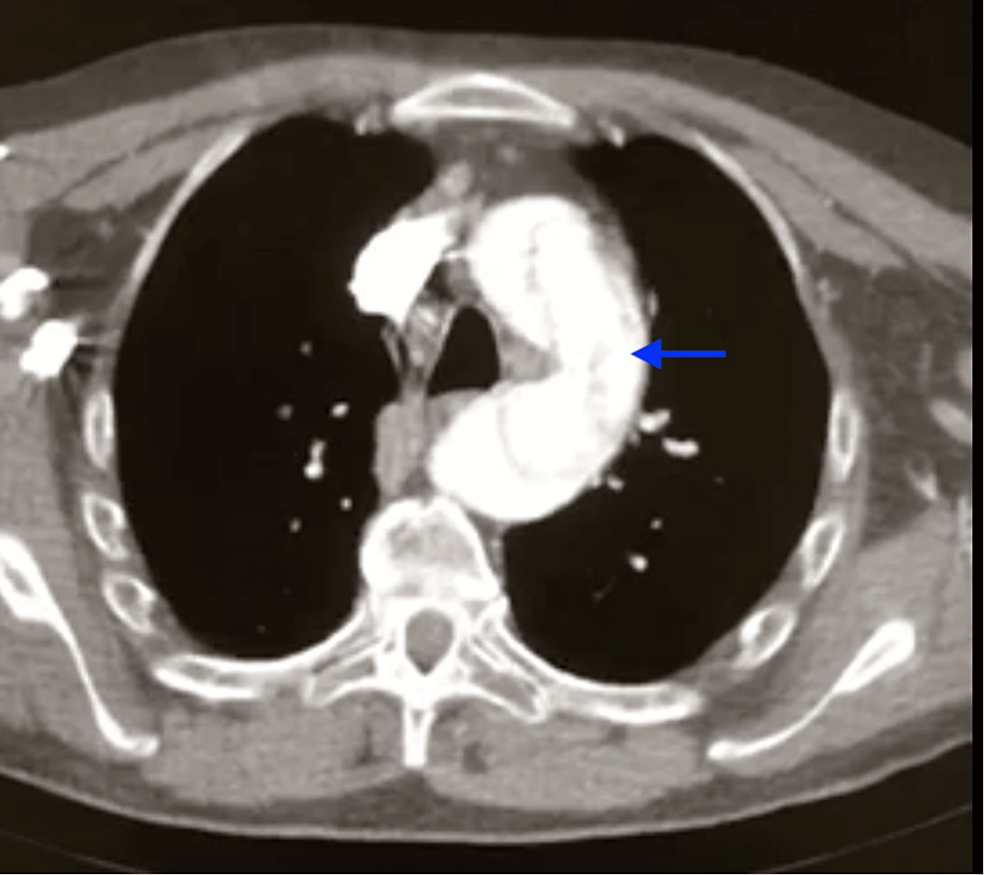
CT angiography showing aortic dissection at the level of the aortic arch; blue arrow points to the false lumen
